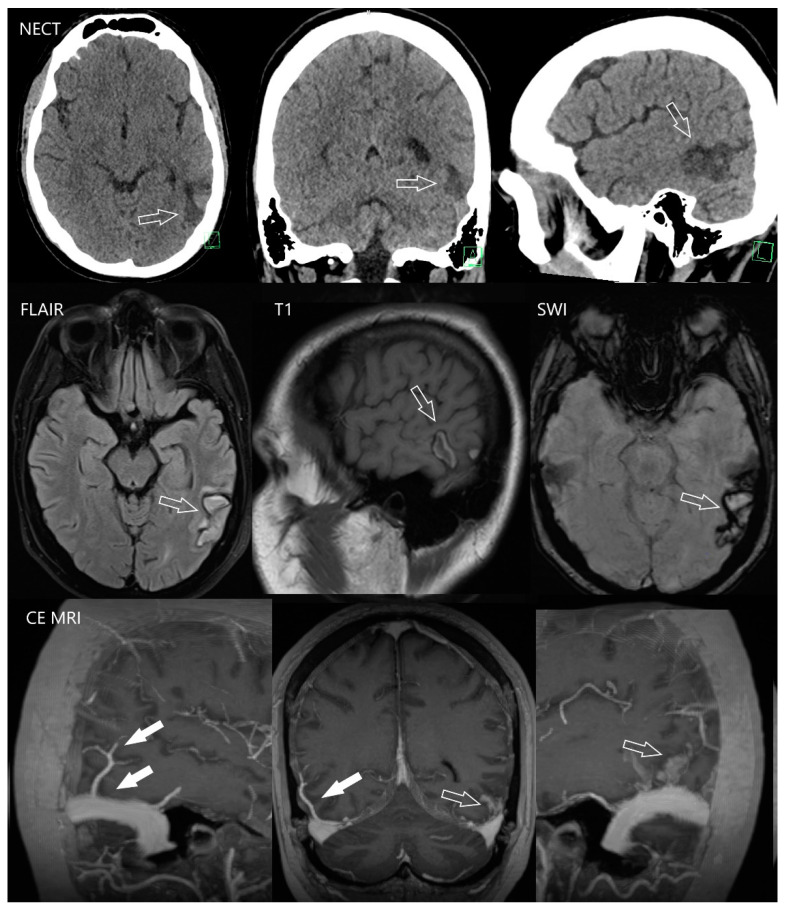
Cerebral venous infarction due to occlusion of the vein of Labbé. 21-year-old male with history of long-term lasting ulcerative colitis treated by vedolizumab and azathioprine. He came to emergency due to the first epilepsy seizure attack in his life. The next relevant data from his history were as follows: Three months before, he underwent embolic occlusion of the femoral artery that was treated by urgent surgery. The source of emboli was not revealed. Two months before, he was treated for acute thrombosis of the femoral vein. NECT (upper row) revealed hypodense region in the posterior aspect of the left temporal lobe (arrows), MRI was indicated to specify the finding. MRI (middle row) depicted the unregular subacute hematoma (arrows) which was suspected from hemorrhagic transformation of venous infarction. On 3D T1 WI (lower row) after gadolinium-based contrast agent application the left vein of Labbé was not present (open arrows). Compare with the right vein of Labbé (full arrows) which has normal appearance.
